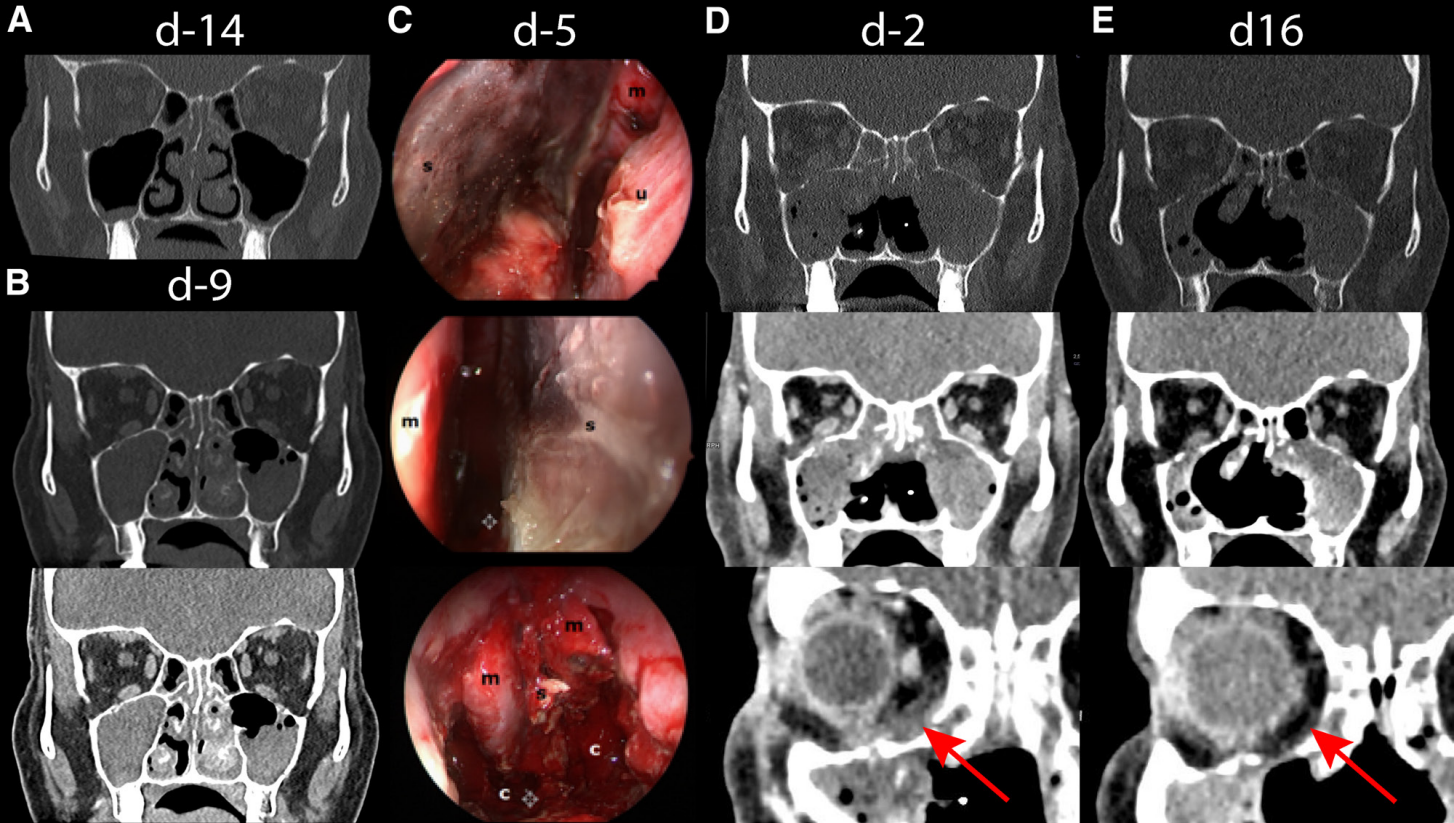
CT scans and intraoperative images. Initial CT scan showing chronic infection of the paranasal sinuses with obstruction of ethmoidal, sphenoidal, and maxillary sinuses 1 d prior to diagnosis of mixed invasive fungal infection with aspergillosis and mucormycosis on day –13 (A). A follow-up CT scan (3 d after classical endonasal ethmoidectomy on day –9) revealed progression of paranasal mucormycosis (B). Several pansinus revisions with median hemimaxillectomy on both sides and subtotal resection of the necrotic nasal septum were performed. The intraoperative images on day –5 (C) show the necrotic nasal septum (s) from both sides and the status after its resection. A postoperative CT scan on day –2 revealed a further progression of paranasal mucormycosis with increased bone affection of the paranasal sinuses and new bilateral infiltration of the orbit (D). Sixteen days after treatment initiation with nivolumab on day 0 complemented by 5 doses of interferon-γ, a CT scan revealed a partial remission with regression of mucormycosis invasion in the sphenoidal and maxillary sinuses (E). c = choanae; CT = computed tomography; m = middle turbinate; u = inferior turbinate.
